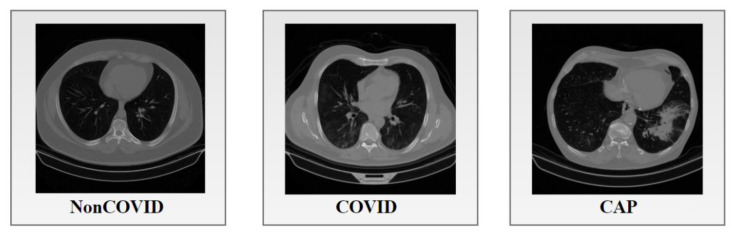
CT images of the three classes of the dataset.
